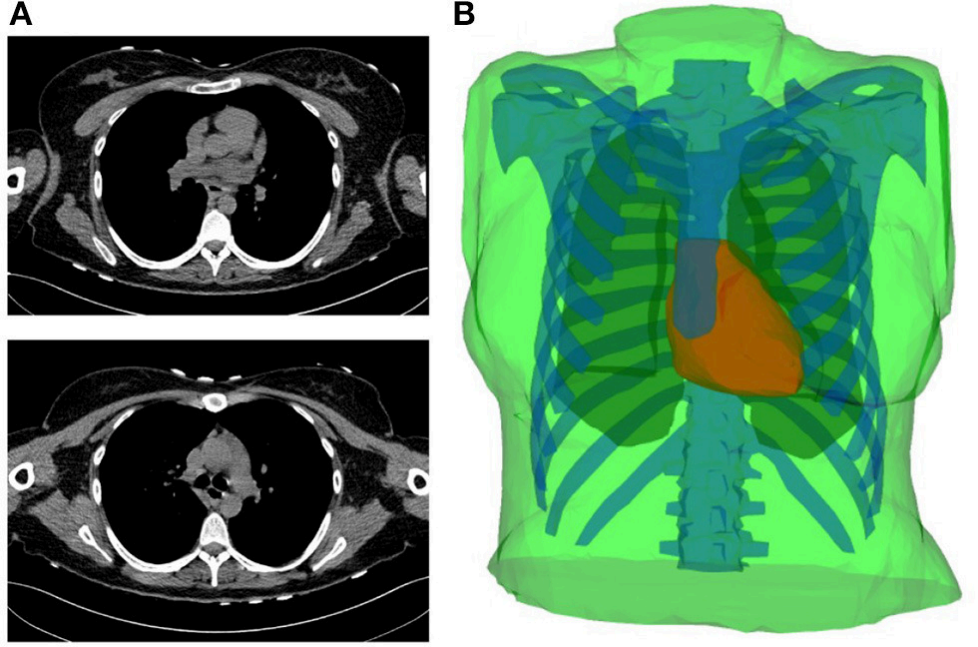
(A) Two slices of the CT-scan images. (B) Torso geometry showing the epicardium (heart-torso interface Σ) (red), lungs (yellow), bones (blue) and torso external boundary Γ_*ext*_ (green).
